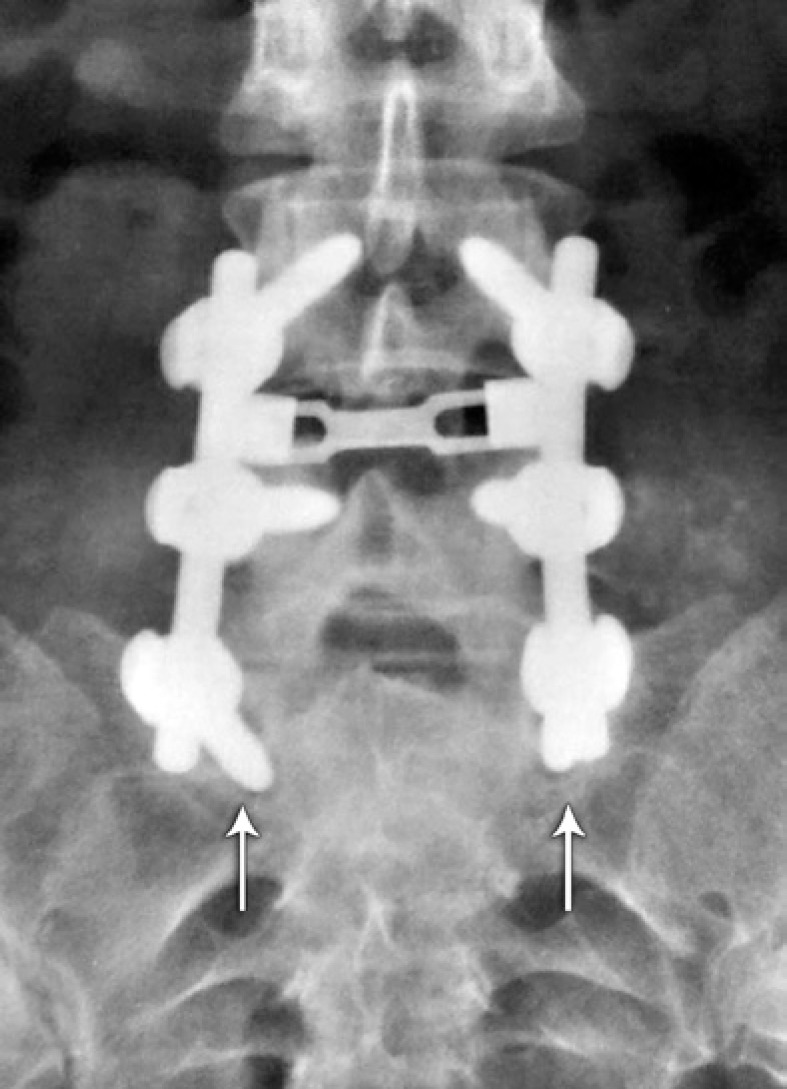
Anteroposterior radiography of the lumbosacral spine, in which pseudoarthrosis is considered due to the presence of osteolysis around screws of S1 (shown by the arrows)
